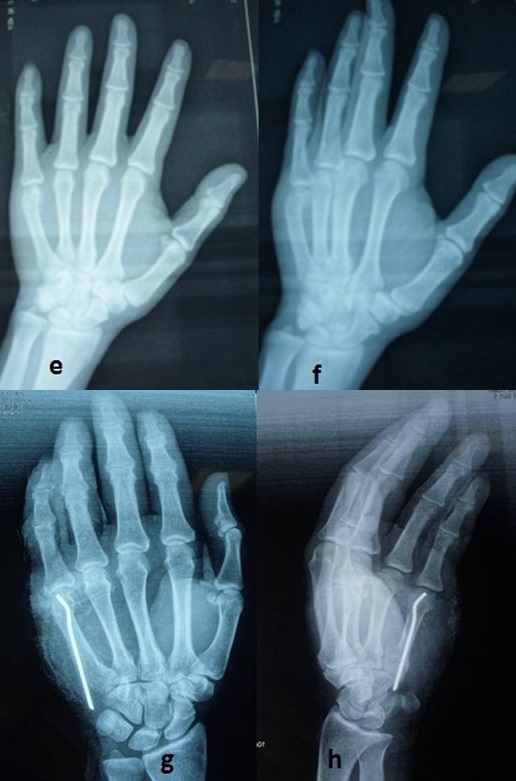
(e,f): Radiograph of a 28 years old male patient with displaced little finger metacarpal neck fracture; (g,h): Postoperative radiograph of the same patient treated with intramedullary nailing
